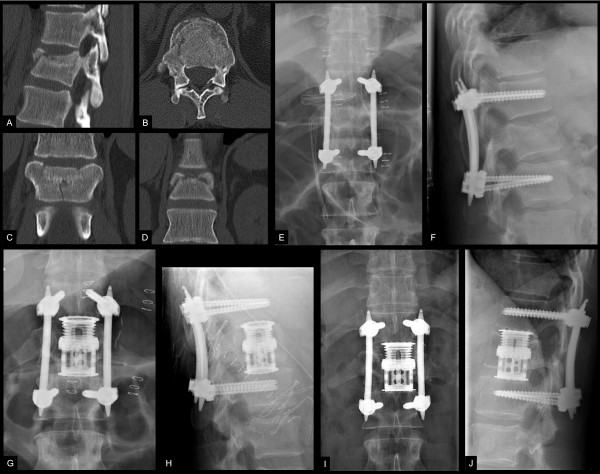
Minimal-invasive percutaneous instrumentation and secondary anterior surgery in a polytraumatized patient with burst fracture of T12. This is a case of a 32 year old male patient following a motor bike accident. The patient suffered from hematopneumothorax, intracapsular rupture of the liver, humeral head fracture and moderate traumatic brain injury resulting in an ISS of 34. Following primary survey and whole body CT-Scan, the patient was transferred to the OR. A chest tube was inserted and the patient was positioned prone for primary stabilization of the type A3.3 fracture of T12 (images A-D). Closed reduction and percutaneous pedicle insertion allowed quick surgery (45 minutes) and limited surgery related injury without substantial blood loss and excessive antigen load as compared to conventional open stabilization (images E-F). After uneventful recovery, definitive anterior surgery using a thoracoscopy assisted approach was performed on day 7 post trauma (images G-H). Follow-up at 24 months shows good operative result of the bisegmental fusion (images I-J).
